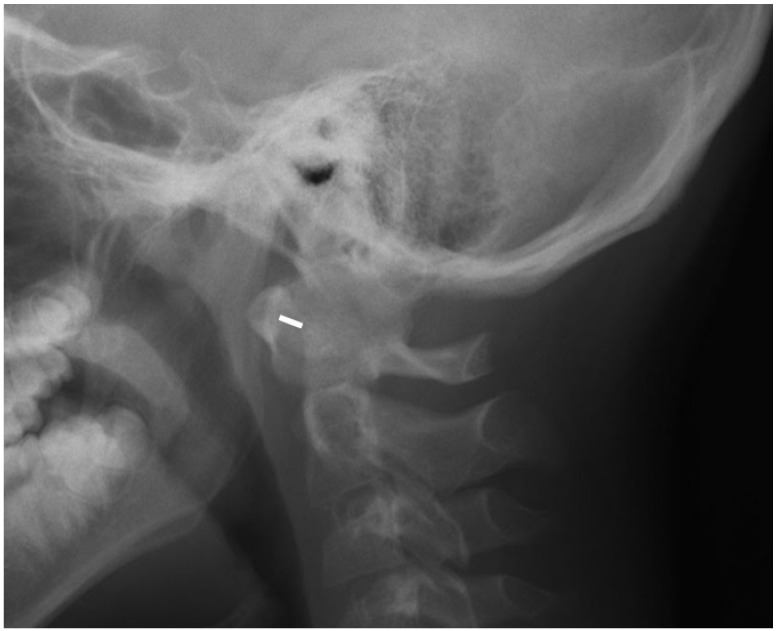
Lateral radiograph in neutral position in a 12-year-old boy (the same patient as in Figure 2) showing anterior atlantoaxial subluxation 6 mm (white line).
